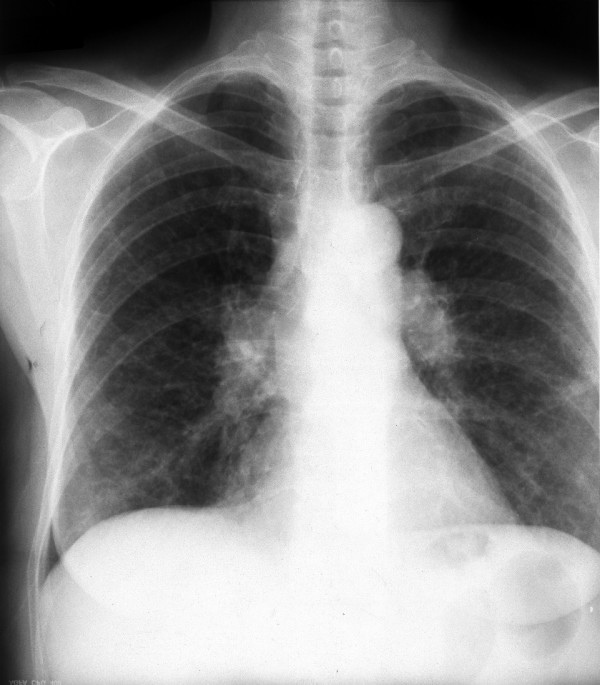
Chest X-ray showing bilateral hilar lymphadenopathy together with lower bilateral interstitial lung densities.
